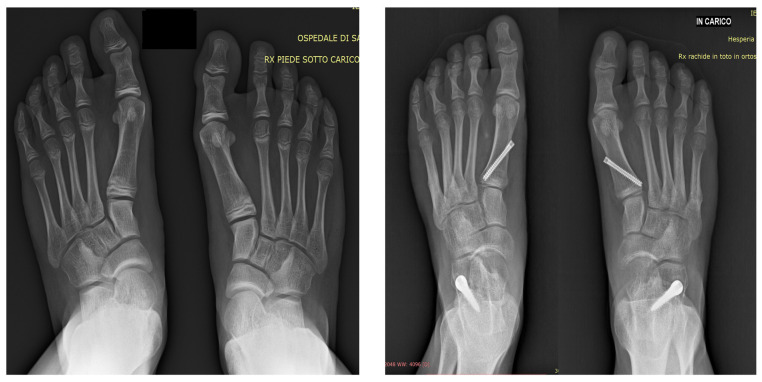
Standing preoperative radiographic appearance of flatfoot with hallux valgus (left) and standing radiographic appearance at two years postoperatively (right).
